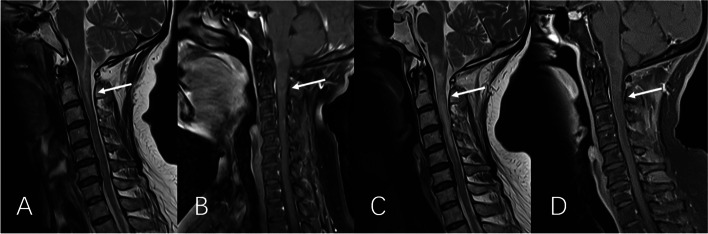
MRI findings of cervical cord. A and B Prior to treatment, MRI showed myelopathyfrom medulla oblongata to spinal cord C3; C and D MRI reexamination showed that the range of myelopathy was enlarged to spinal cord C5. White arrow indicates the lesion. A and C are T2-weighted images, while B and D are T1-weighted images
